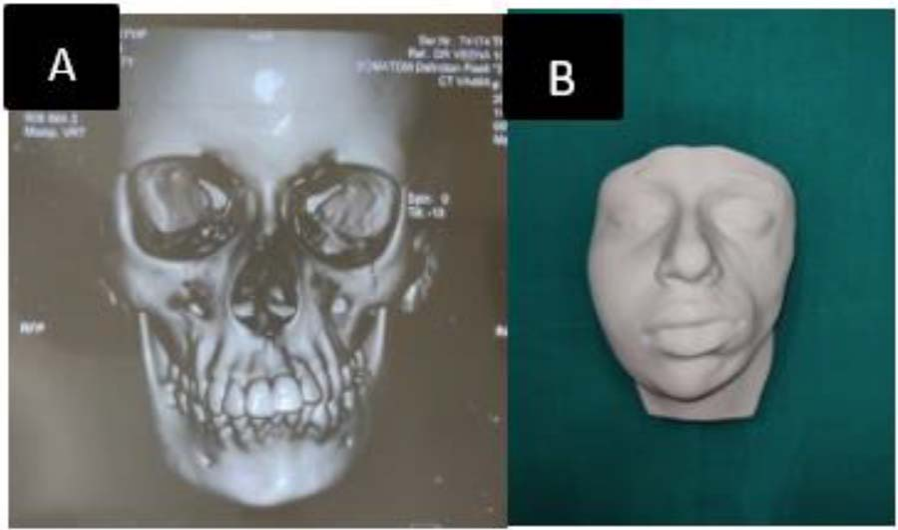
(A) Preoperative CT showing shortened left hemimandible. (B) 3 D model.
